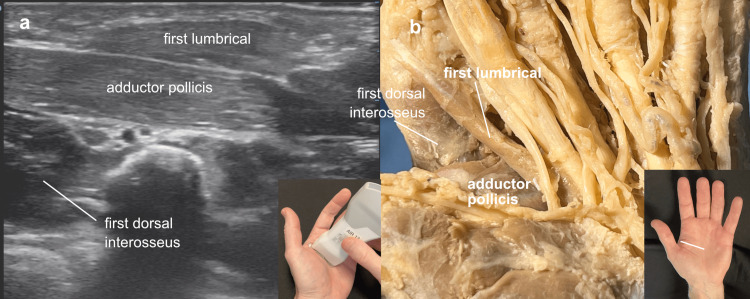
Adductor orientation Adductor orientation: (a) Ultrasound view over the palmar first webspace of a left hand, the probe marker directed toward the thumb (left) for easier correlation with the cadaver view, demonstrating long axis views of the adductor pollicis and first lumbrical muscles and a short axis view of the first dorsal interosseus muscle, (b) cadaver demonstration of the same structural relationships.
